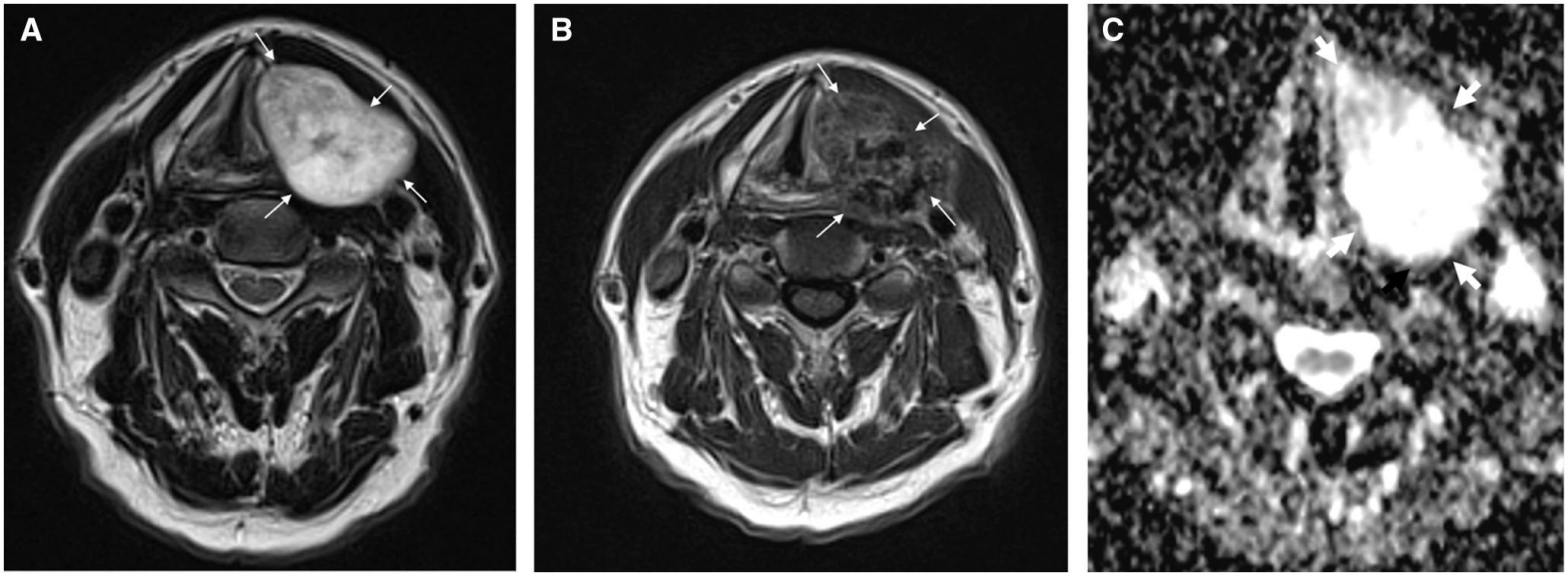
Left thyroid lamina chondrosarcoma. (A) Axial T2-weighted MRI shows a predominantly high signal solid mass replacing the left thyroid cartilage (short thin white arrows). (B) Axial non-fat-suppressed post-contrast T1-weighted MRI demonstrates heterogeneous contrast enhancement. (C) ADC map demonstrates no restricted diffusion.
